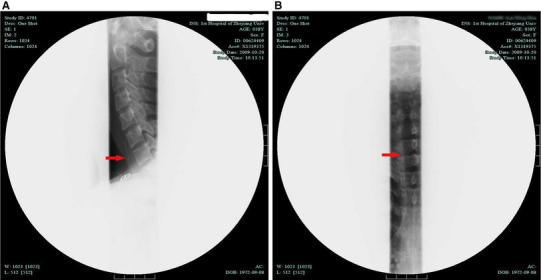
A plain radiograph showing a linear hyperdense area (*arrow*) in front of the C7 and T1 bodies: a sagittal image, b coronal image
